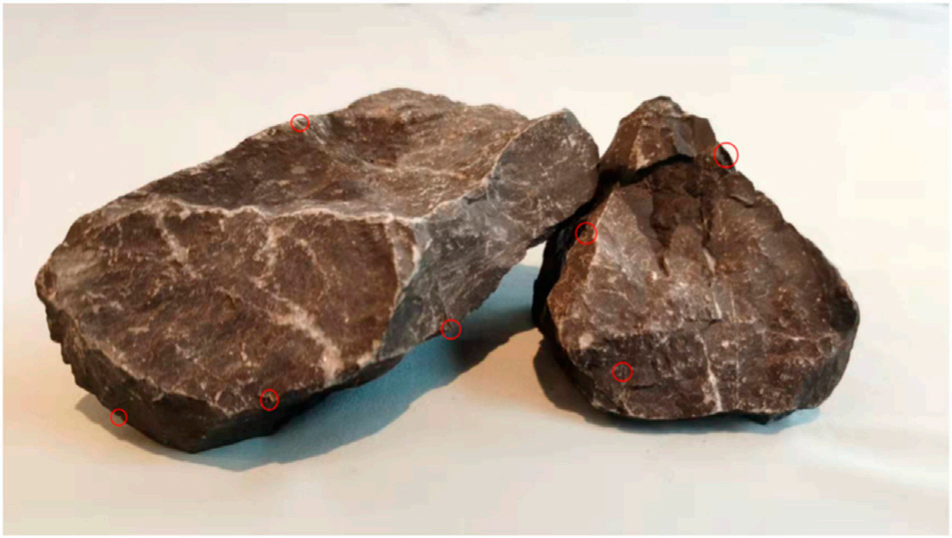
There are many small indentations on the surface of the aquarium stones (small red circles). In their MRI image, those indentations that meet the CAF standard are used as characteristic areas, to improve the measurement accuracy.
